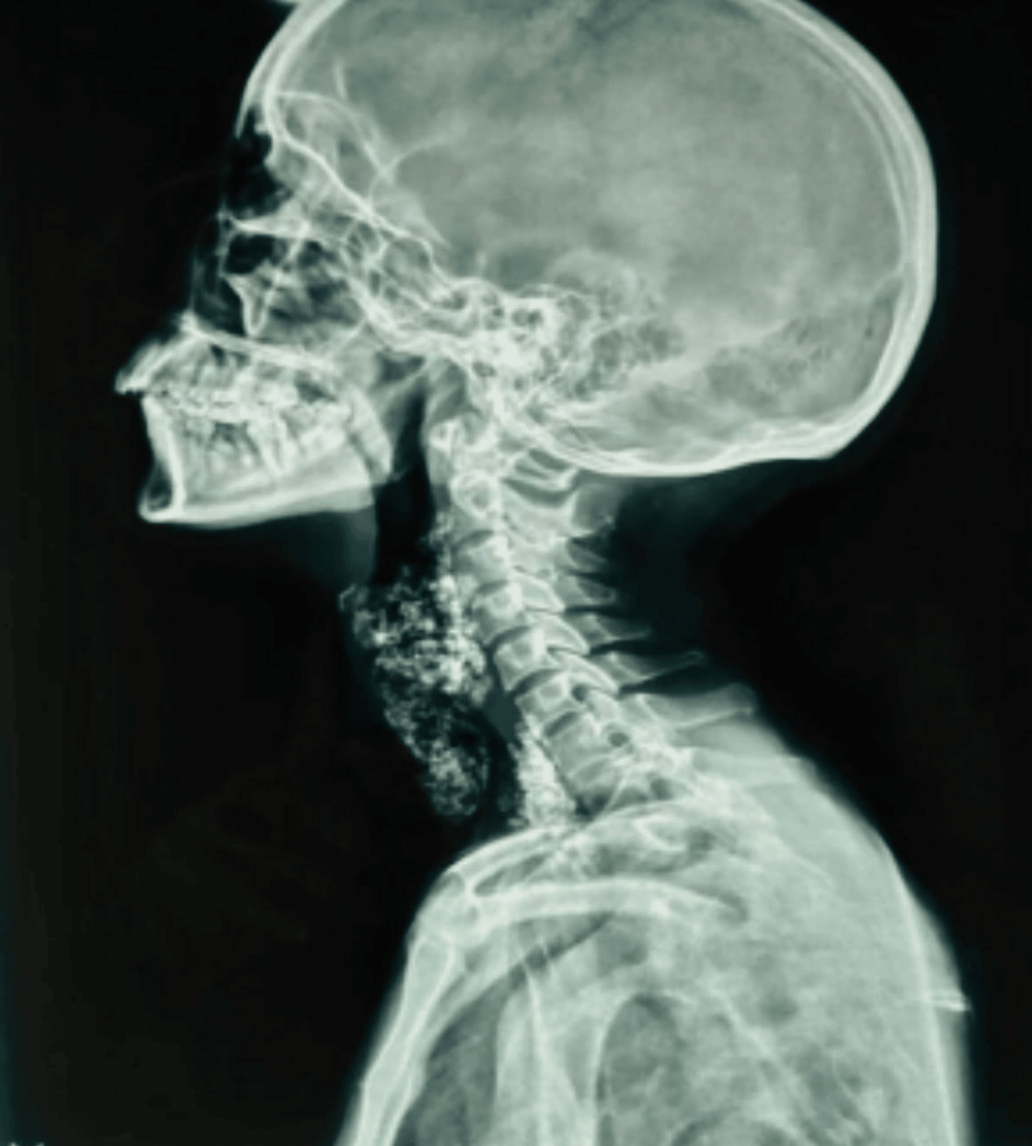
X-ray lateral view of the neck showing diffuse pleomorphic calcifications in the pretracheal, infrahyoid location, extending from C2 to T2 and beyond the thoracic inlet
